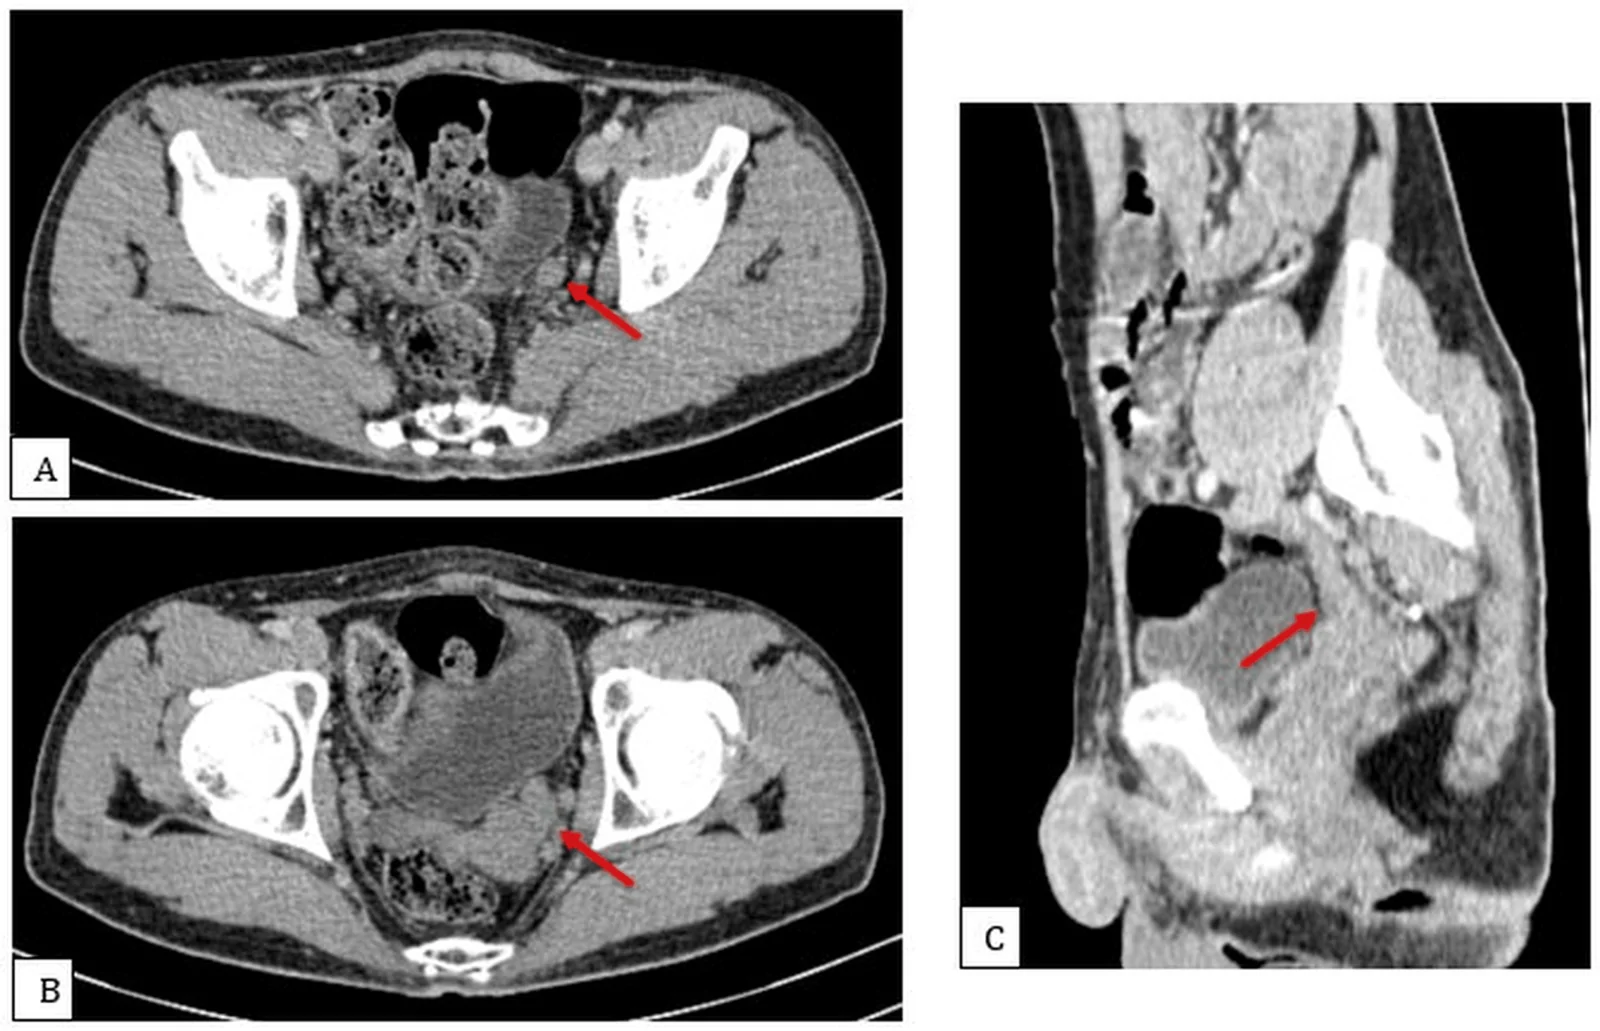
Contrast-enhanced computed tomography (CT) images. (A) Axial image of the pelvis demonstrating the left ureter (red arrow). (B) Axial image and (C) oblique sagittal reconstruction showing ectopic insertion of the left ureter into the seminal vesicle cystic lesion (red arrow).
